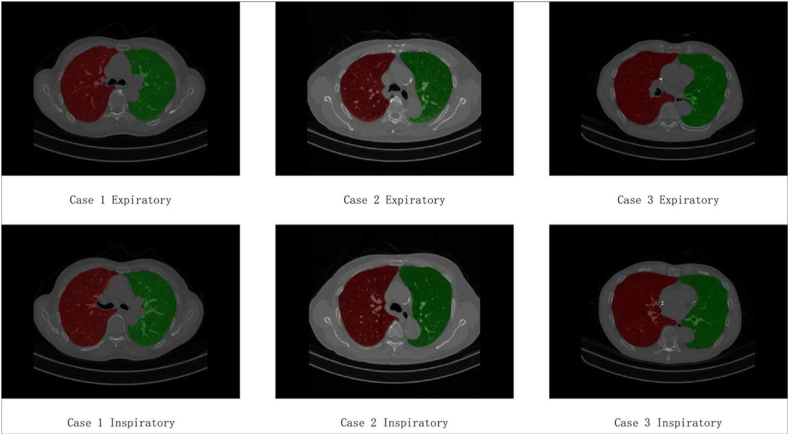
The typical lung parenchyma mask images of inspiratory and expiratory chest low-dose CT images.
